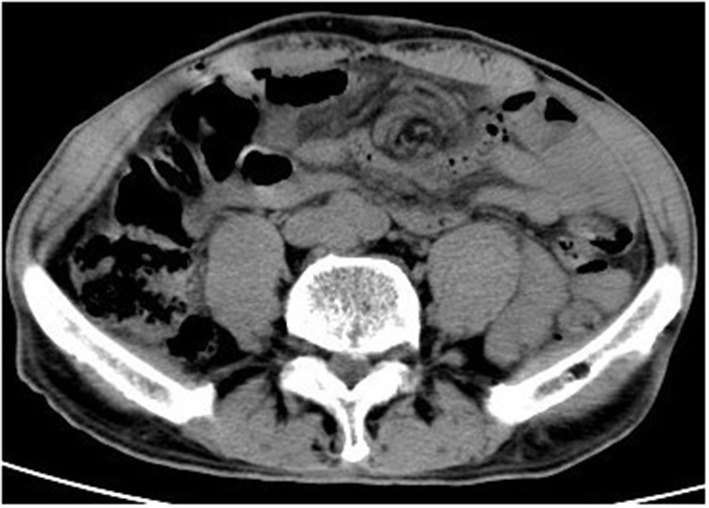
CT of the abdomen on re-admission, the red arrow indicates the “vortex sign” that marks volvulus.
